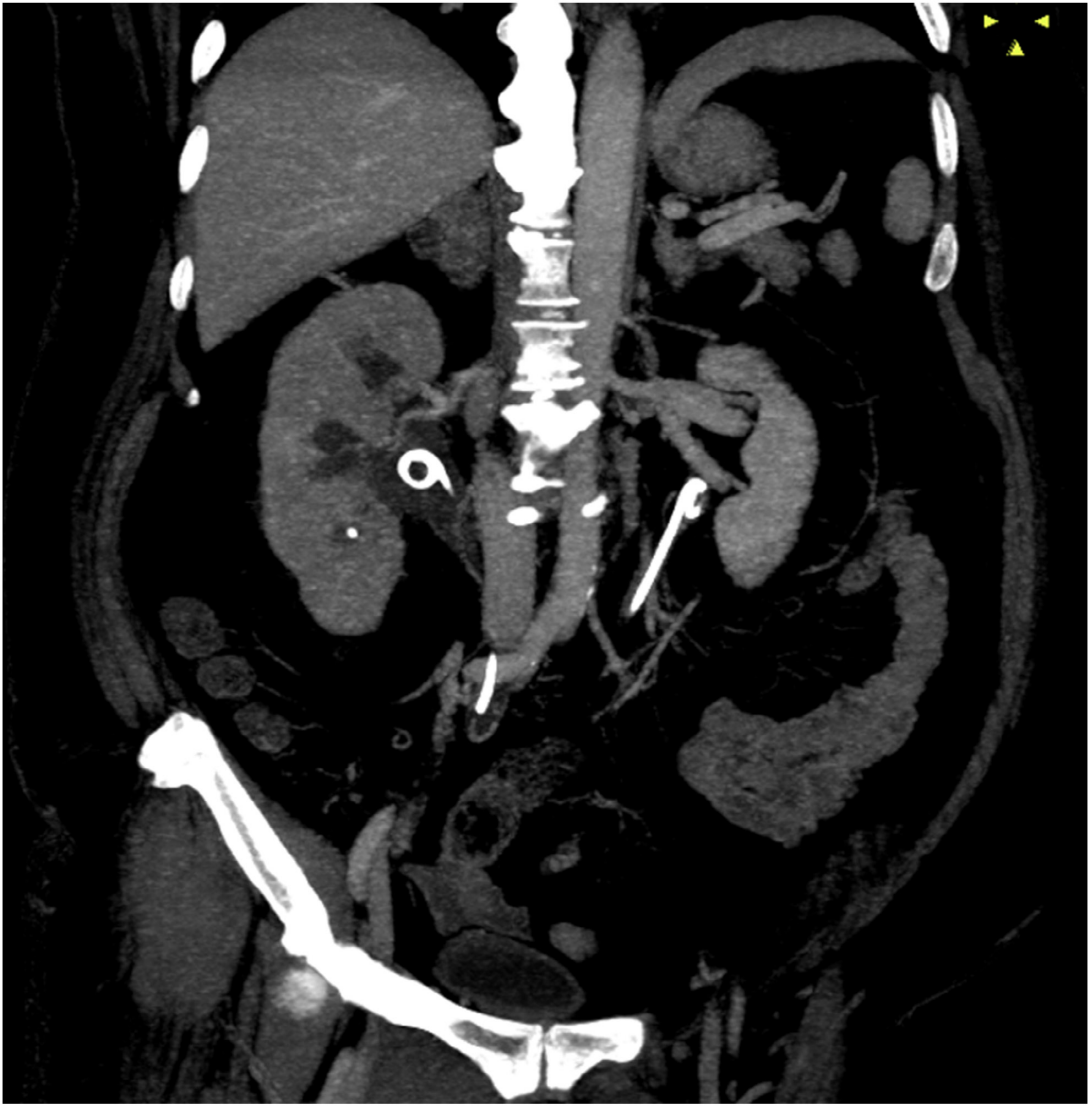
Computed tomography, coronal view, showing two double‐J stents in the right and left renal pelvis.
